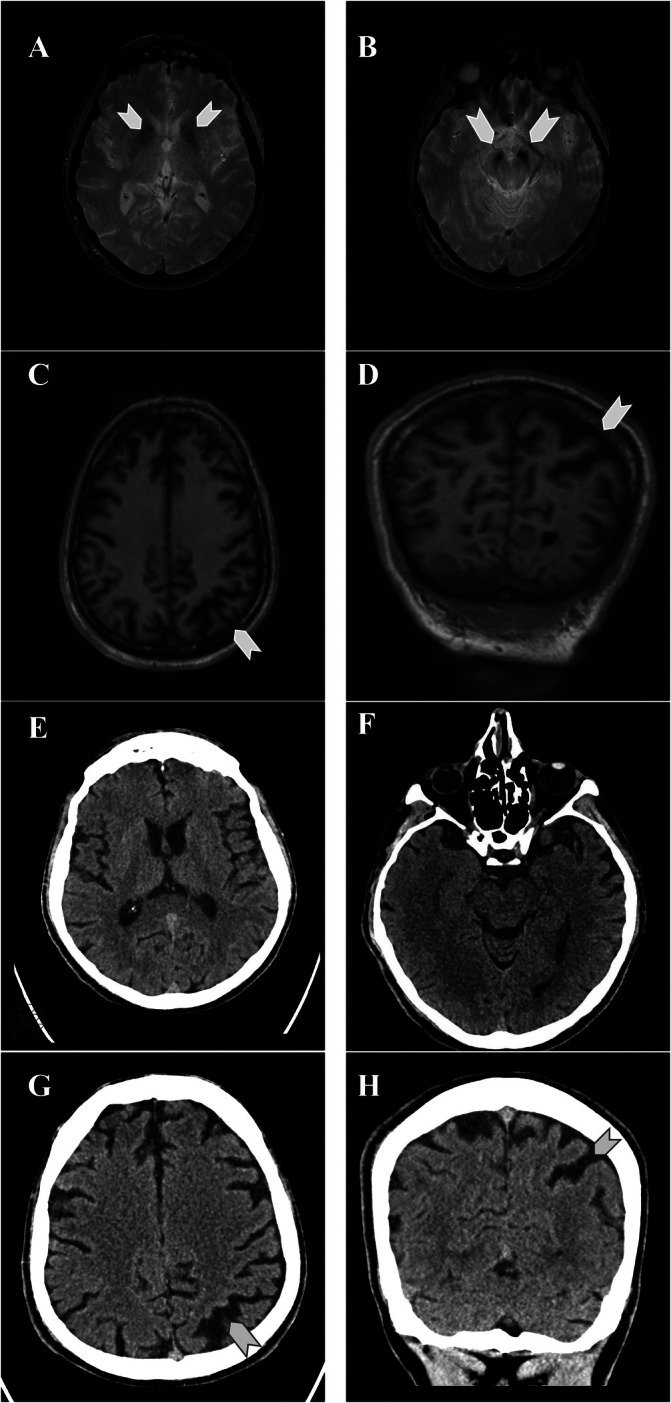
Brain MRI and CT of the patient at 57 years. Axial‐gradient‐recalled‐echo‐T2*‐weighted images demonstrate bilateral hypointensities in the substantia nigra (A) and globi pallidi (B), likely related to paramagnetic deposits. 3D‐T1‐weighted axial reconstruction (C) shows diffuse cortical atrophy mostly prevalent in the parietal regions. Parietal atrophy is also evident in the coronal section (D). SWI (susceptibility Weighted Imaging) sequence could not be obtained due to motion artifacts. Axial CT brain scan shows absence of calcifications in the basal ganglia (E) and substantia nigra (F). Cortical atrophy is visible on axial (G) and coronal reconstruction images (H).
